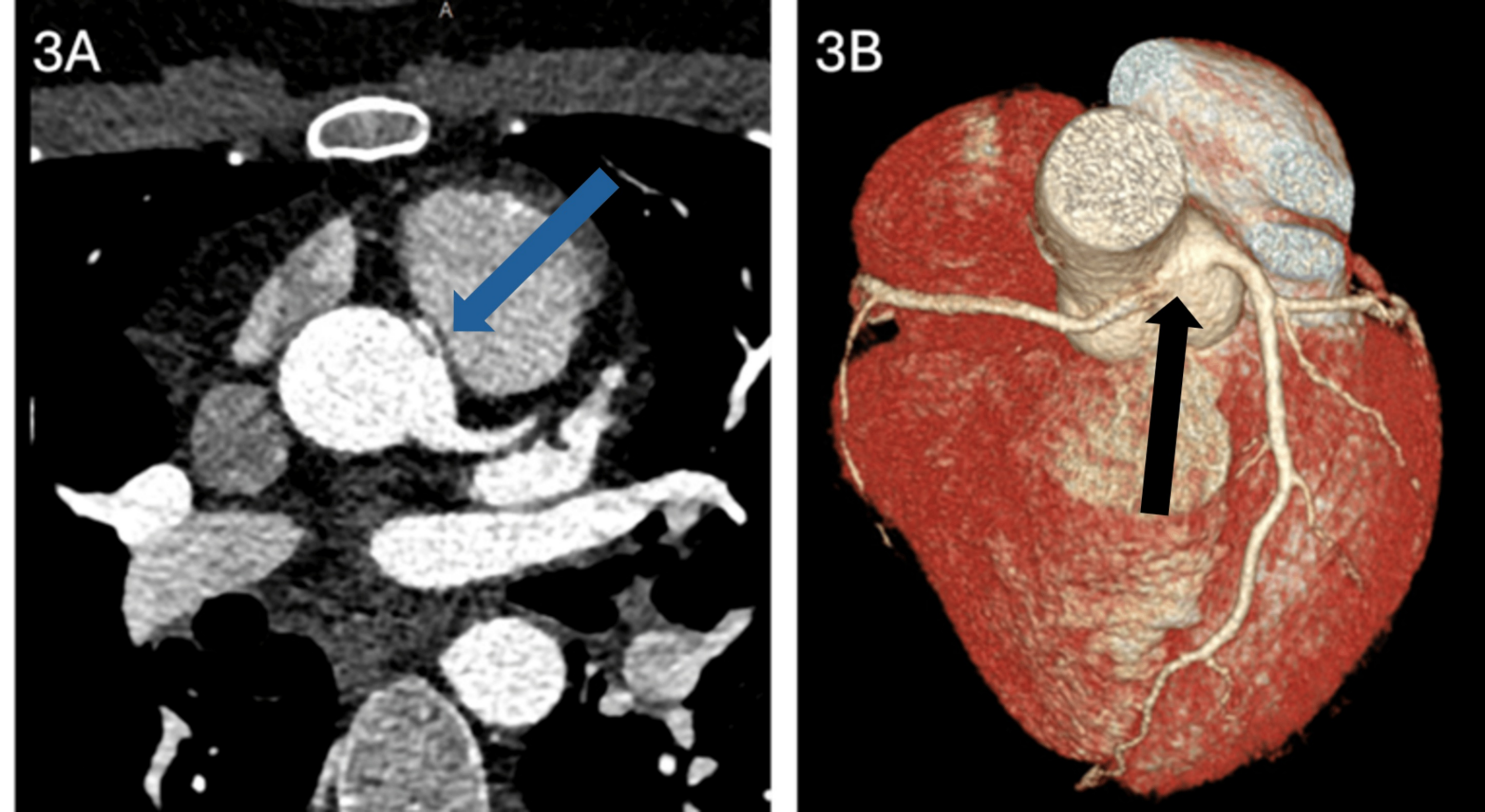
Case 3 Imaging (A) Cardiac computed tomography angiography (CTA) cross-view showing right coronary artery (RCA) originating from the left aortic sinus (blue arrow). (B) Three-dimensional reconstruction image showing the RCA course anterior to the ascending aorta (black arrow). Image credit for B: Dr. Malek Othman.
